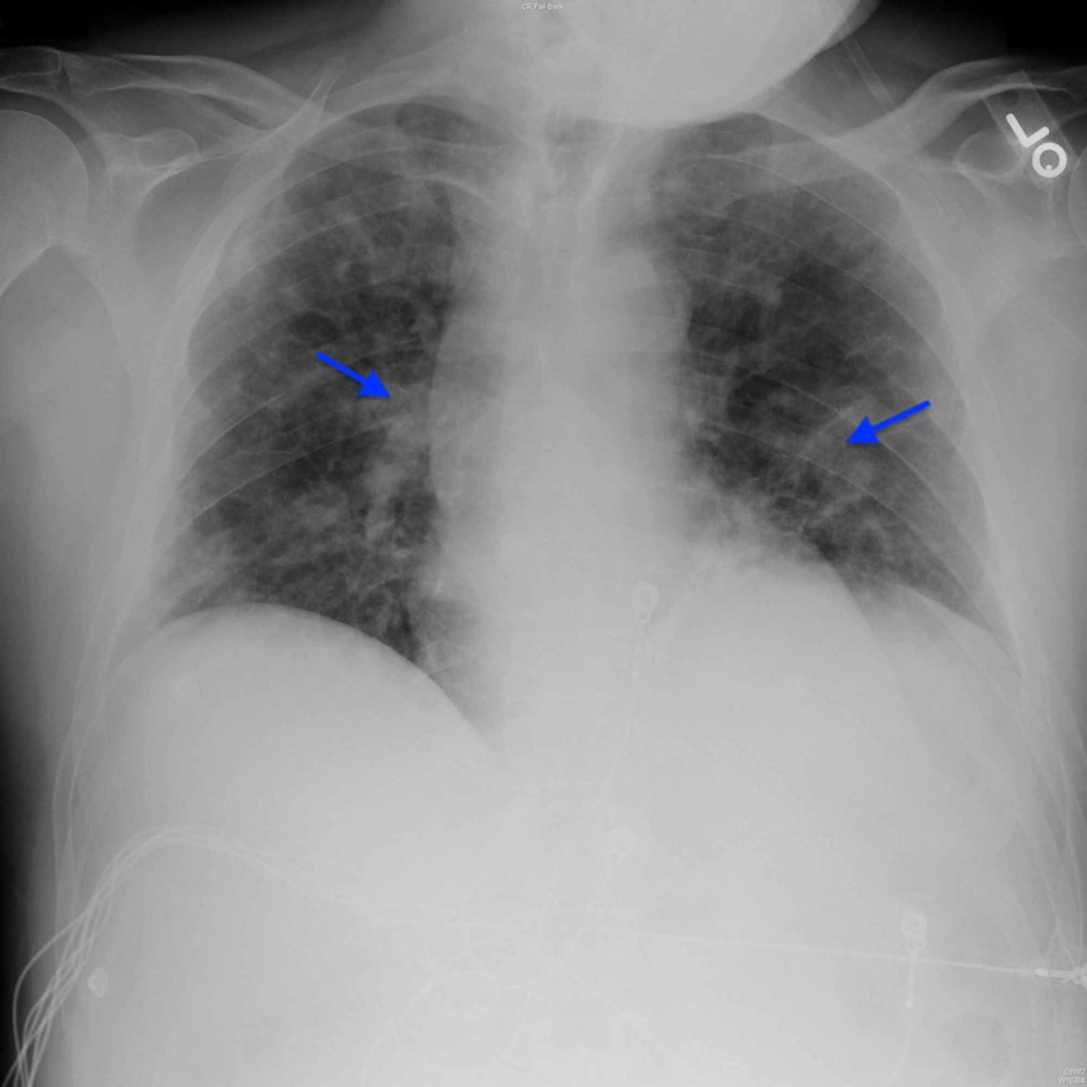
Chest radiograph on day 12 demonstrating stable patchy bilateral airspace opacities with superimposed interstitial thickening (arrows). Cardiomediastinal silhouette and osseous structures are unremarkable.
